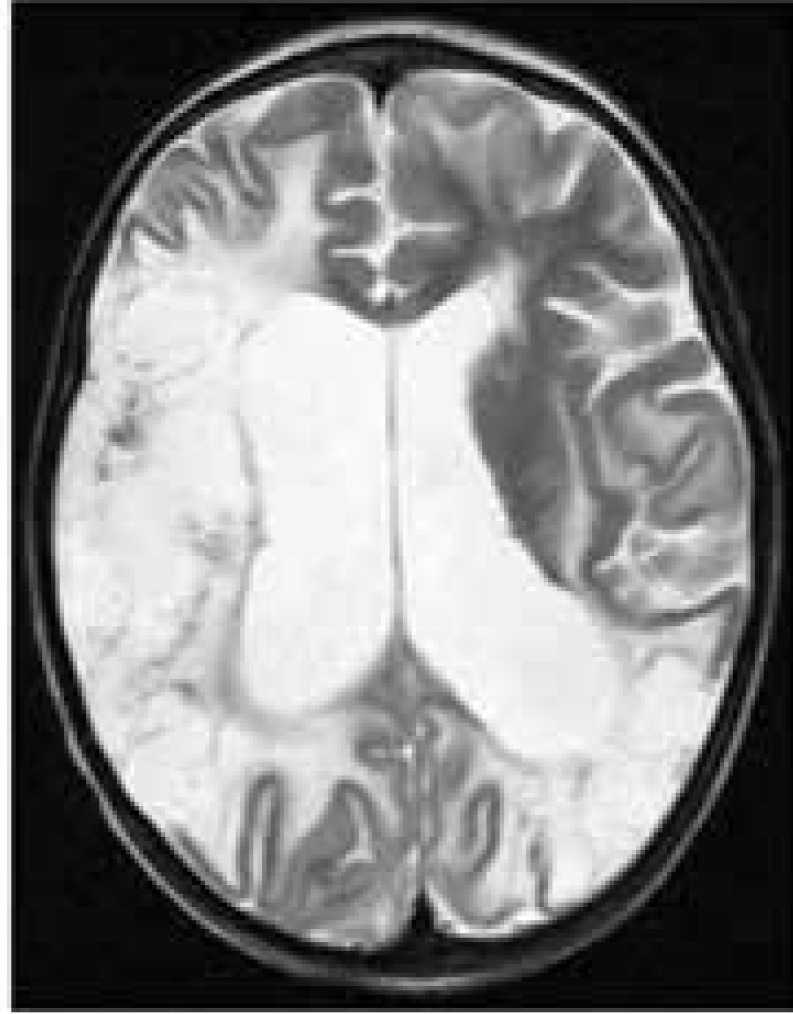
Patient’s brain MRI on the last admission
